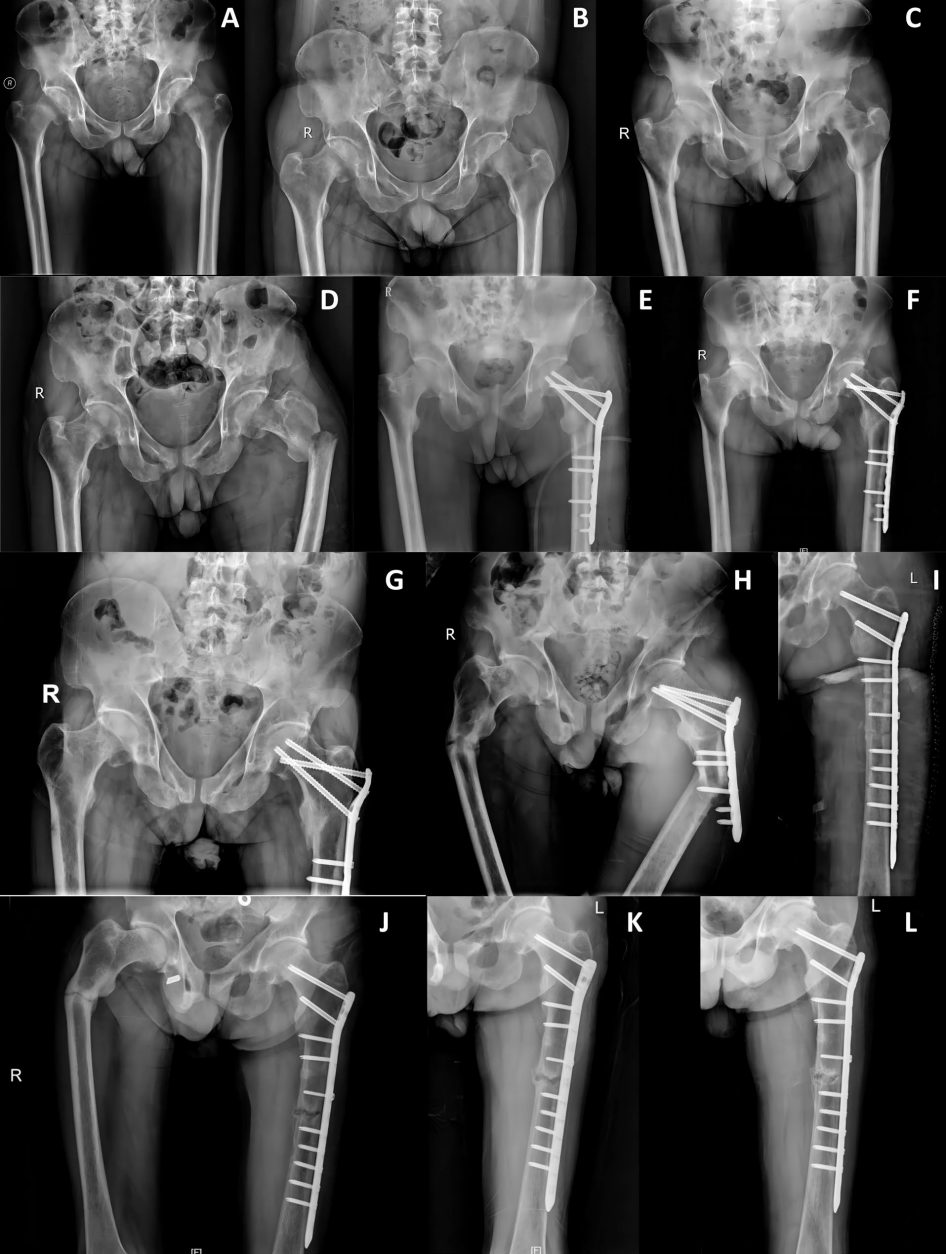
X-rays of the pelvis at different times. A Bilateral fractures of the femoral neck occurred in June 2015. B The previous fracture has not healed and is more displaced than before in September 2016. C The fracture is slightly displaced relative to B and remains unhealed in September 2017. D The fracture of the neck of the femur did not fully heal and in March 2018, and a left subrotunda fracture of the femur occurred. E Bilateral femoral neck fractures showed healing after post-op in March 2018. F The left intertrochanteric fracture of the femur gradually healed, but the plate on the femoral side showed signs of loosening in June 2019. G The subtrochanteric fracture has healed, but the right side of the proximal femur showed signs of fracture on the lateral cortex in September 2021. H Bilateral fractures of the proximal femur. Notably, the original subtrochanteric fracture on the left side has healed and the plate has loosened, resulting in the development of a new fracture at the femoral stem in March 2024. I The left proximal femur fracture with good alignment of the fracture ends after internal fixation with an incised reduction plate was given in March 2024. J, K, L The fractured end of the left femoral stem gradually began to form a bone scab in May, June, and August 2024
